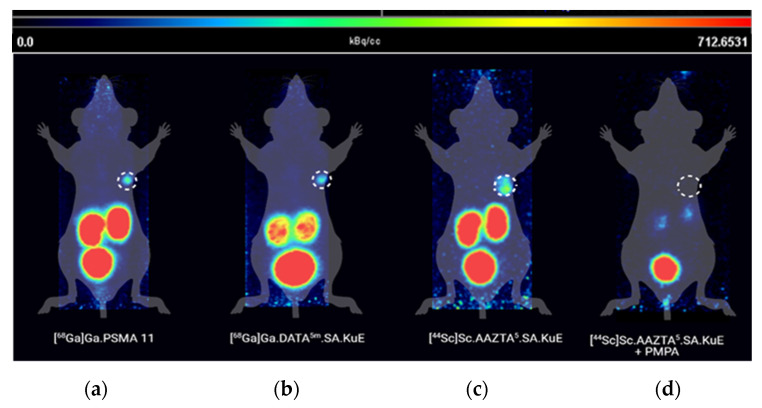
Maximum intensity projections of µPET scans 1 h p.i. of (a) [^68^Ga]Ga-PSMA-11, (b) [^68^Ga]Ga-DATA^5m^.SA.KuE, (c) [^44^Sc]Sc-AAZTA^5^.SA.KuE and (d) co-injection of [^44^Sc]Sc-AZTA^5^.SA.KuE and PMPA (tumor circled).
